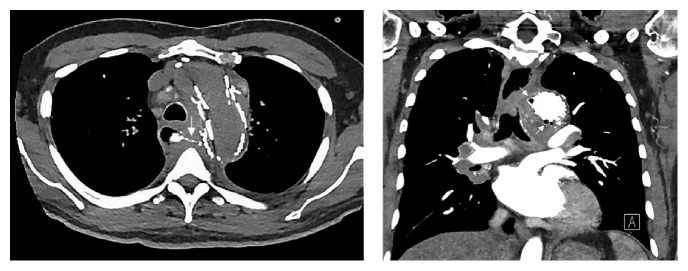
CT angiography axial view showing linear high-density contrast collection extending from esophageal wall towards the aortic graft representing retained barium contrast from the patient's recent upper gastrointestinal series visualizing fistulous tract and the coronal view showing multiple foci of gas or air bubbles adjacent to the patient's aortic stent graft.
